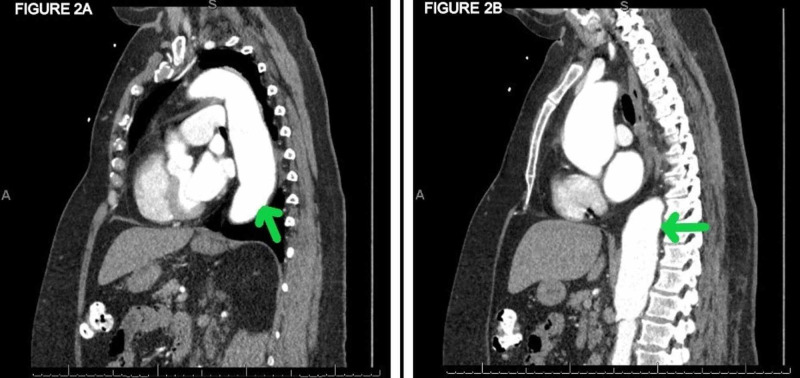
Initial CT abdomen demonstrating a (A) thoracic and (B) abdominal aortic aneurysm Note the fusiform aneurysm of the mid to distal descending thoracic aorta that measured up to 4.7 x 4.5 cm, and fusiform aneurysm of the suprarenal abdominal aorta measuring up to 4.2 x 4.1 cm.
